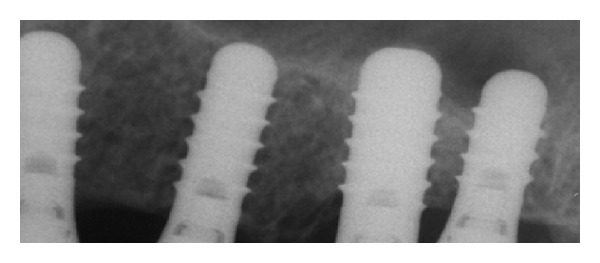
Postoperative radiograph of the left maxillary quadrant four months after the placement of three implants with better initial stability medially and distally to the first implant showing the new sinus floor with all the implants appropriately positioned and inclined.
